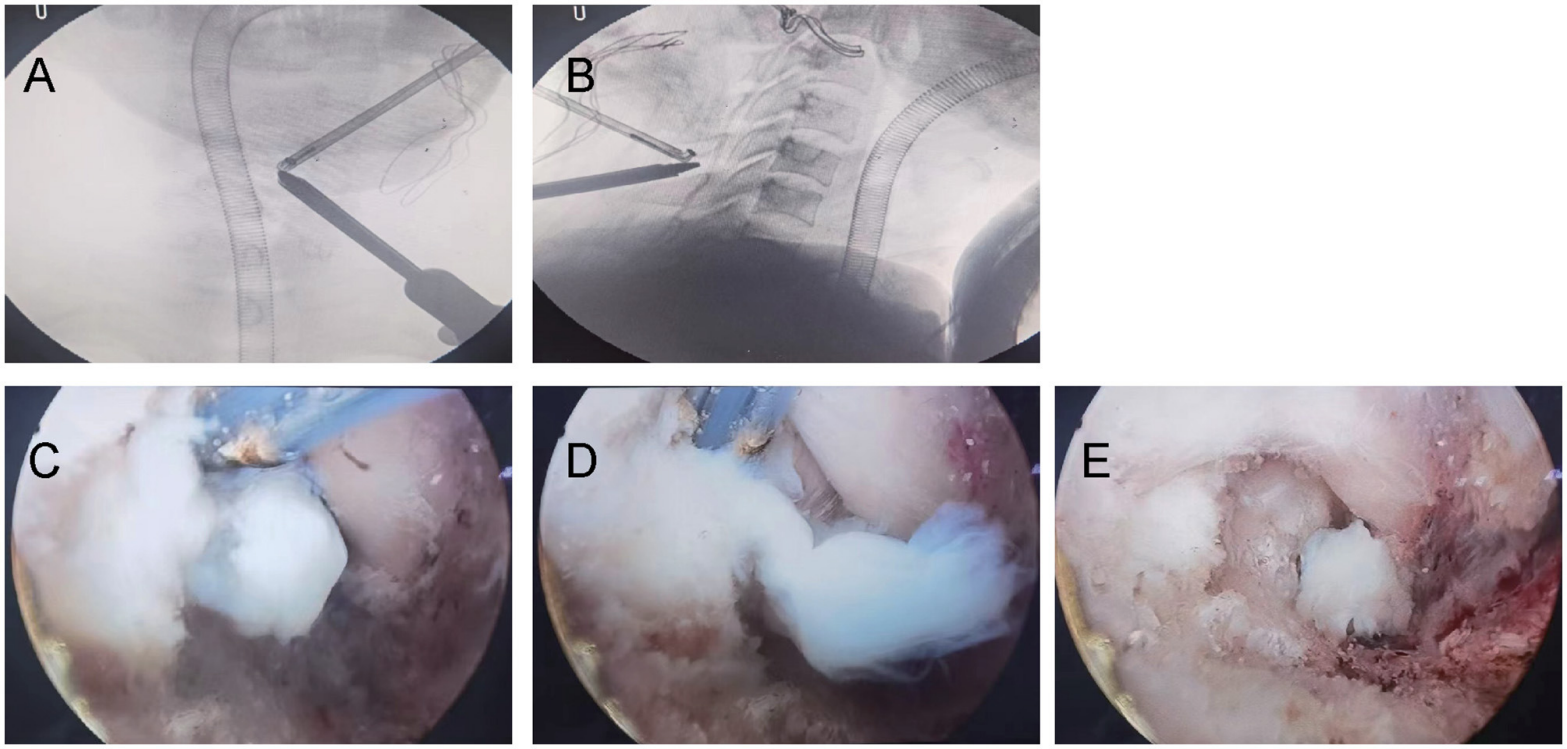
(A, B) Radiographic anteroposterior represented the locations of viewing and working portals. (C–E) Diskectomy was performed.
